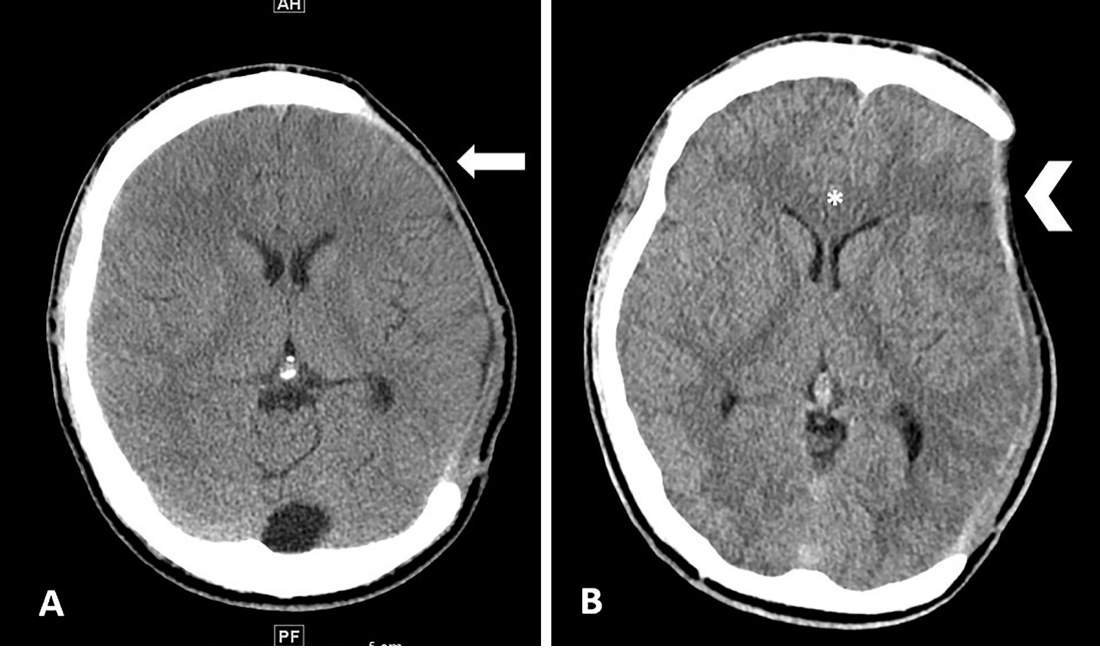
Non-contrast axial head computed tomography (CT) image demonstrating sunken flap and midline shift. Image A is from seven weeks prior to patient’s emergency department (ED) presentation. He is status post left hemicraniectomy. His CT demonstrates a normal-appearing cranioplasty flap (arrow). In comparison, image B is from the day of patient’s ED presentation demonstrating flattening and depression at the area of skull defect (arrowhead) with a corresponding 5 millimeter left-to-right midline shift (star) concerning for paradoxical herniation.
